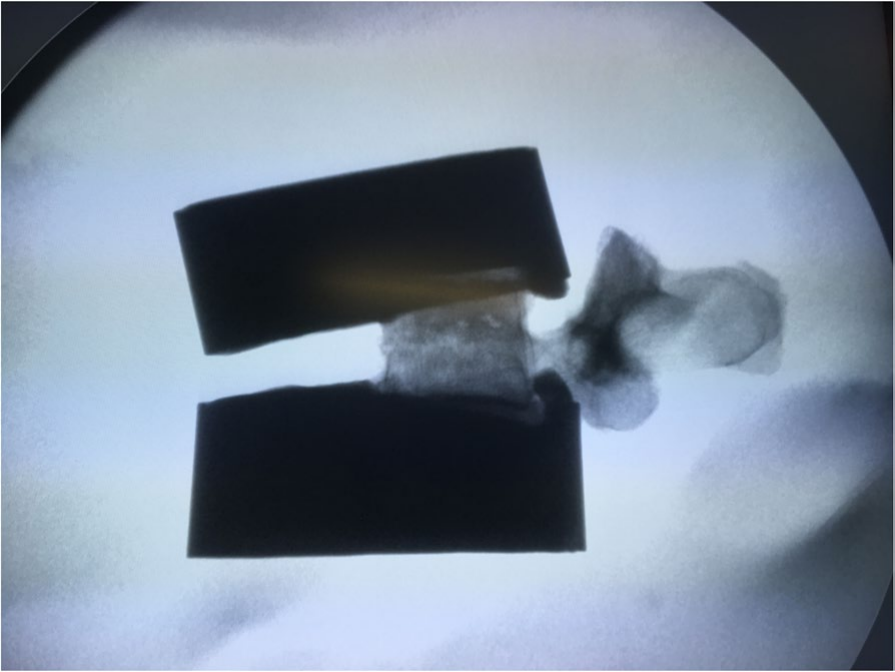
Example of radiograph after fracture generation, which shows a vertebral compression fracture
